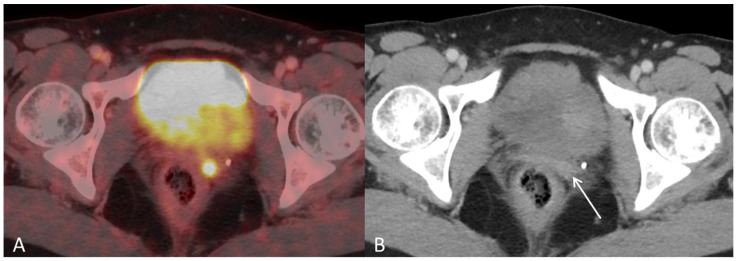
(A) Baseline axial PET/CT fused and (B) axial CT shows a small metabolic active hyperdense tumour located in the left vaginal corner, verified by biopsy as representing a metastasis from endometrial hepatoid adenocarcinoma ((B), arrow).
